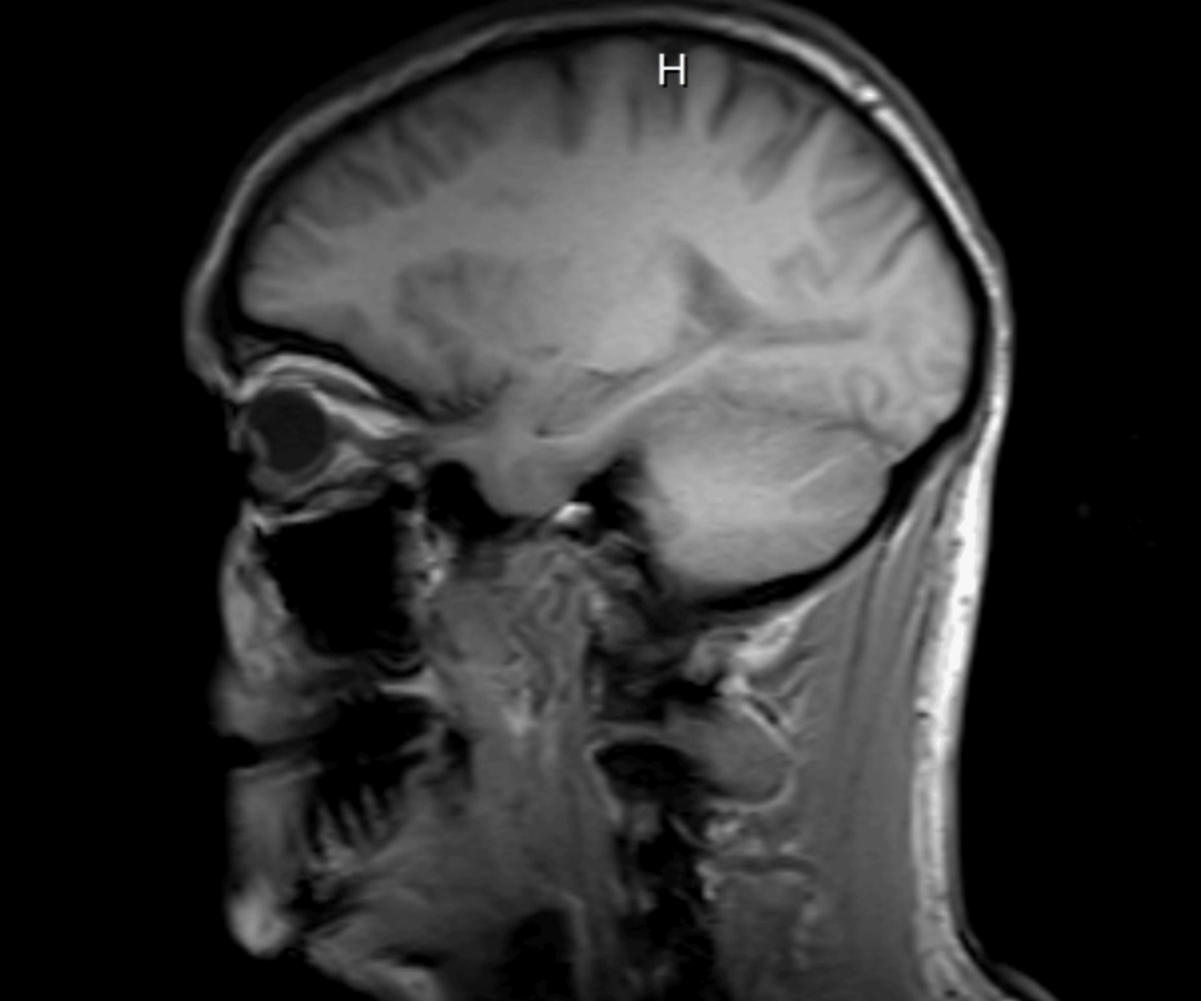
MRI sagittal view T1-weighted. No acute intracranial process.
